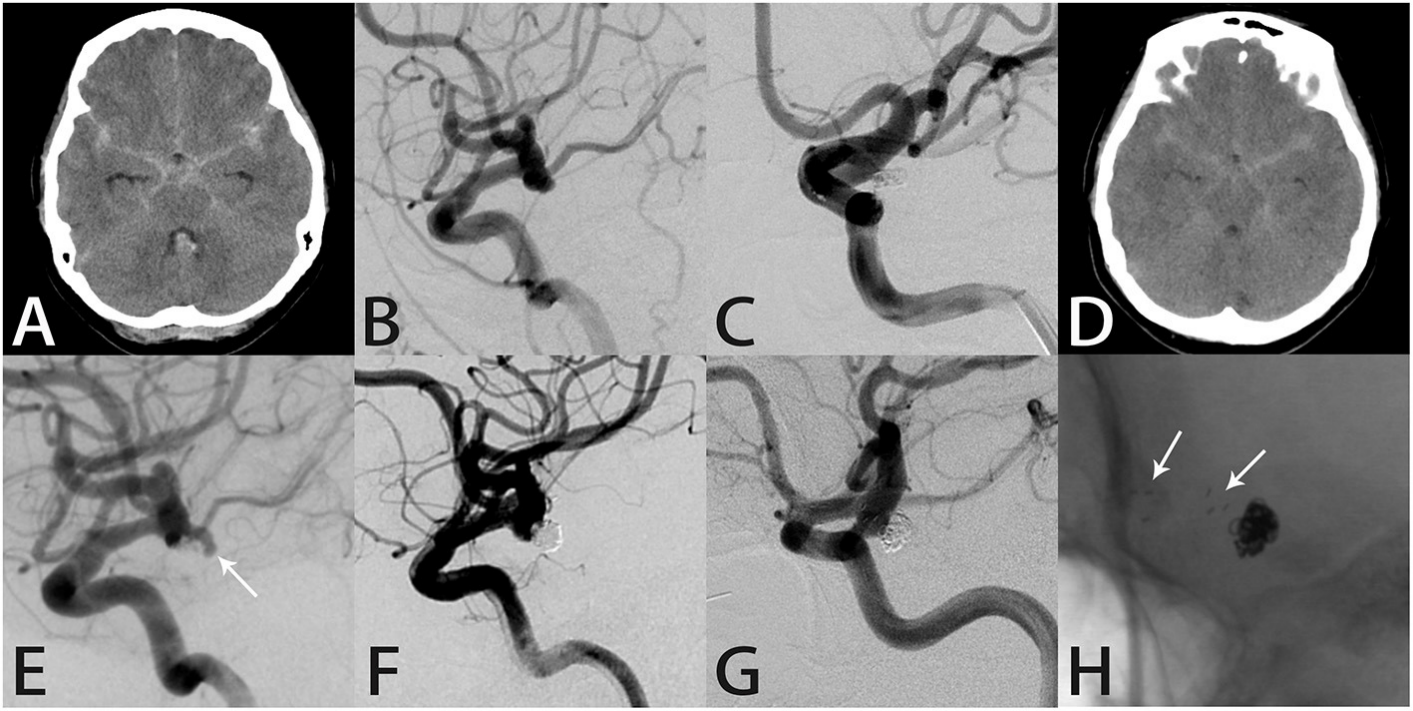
Images from a 51-year-old woman with a left posterior communicating artery aneurysm (case 9). (A) Computed tomography showed subarachnoid hemorrhage in the brain basal cistern, lateral fissure cistern, longitudinal fissure cistern, and ambient cistern. (B) Angiography showed a left posterior communicating artery aneurysm. (C) The aneurysm was occluded completely after coil embolization. (D) Computed tomography showed subarachnoid hemorrhage in the lateral fissure cistern and sulcus. (E) Angiography showed recurrence of the left posterior communicating artery aneurysm. (F) Angiography immediately after re-treatment showed RR class II occlusion. (G) Follow-up angiography 12 months later showed the aneurysm remained RR class II occlusion. (H) Intraprocedural angiography showed the Atlas stent remained stable and the three radiopaque markers (white arrows) could be seen at the proximal and distal ends of the Atlas stent (3.0 × 21 mm).
